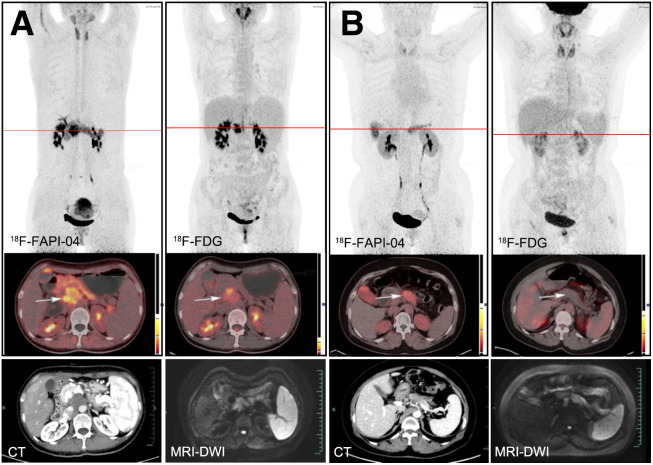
Typical PET (top), PET/CT (middle), and CT and MR (bottom) images of primary tumor obtained using 2 tracers in representative patients (A and B). Tumor is marked by arrows. DWI = diffusion-weighted imaging.
